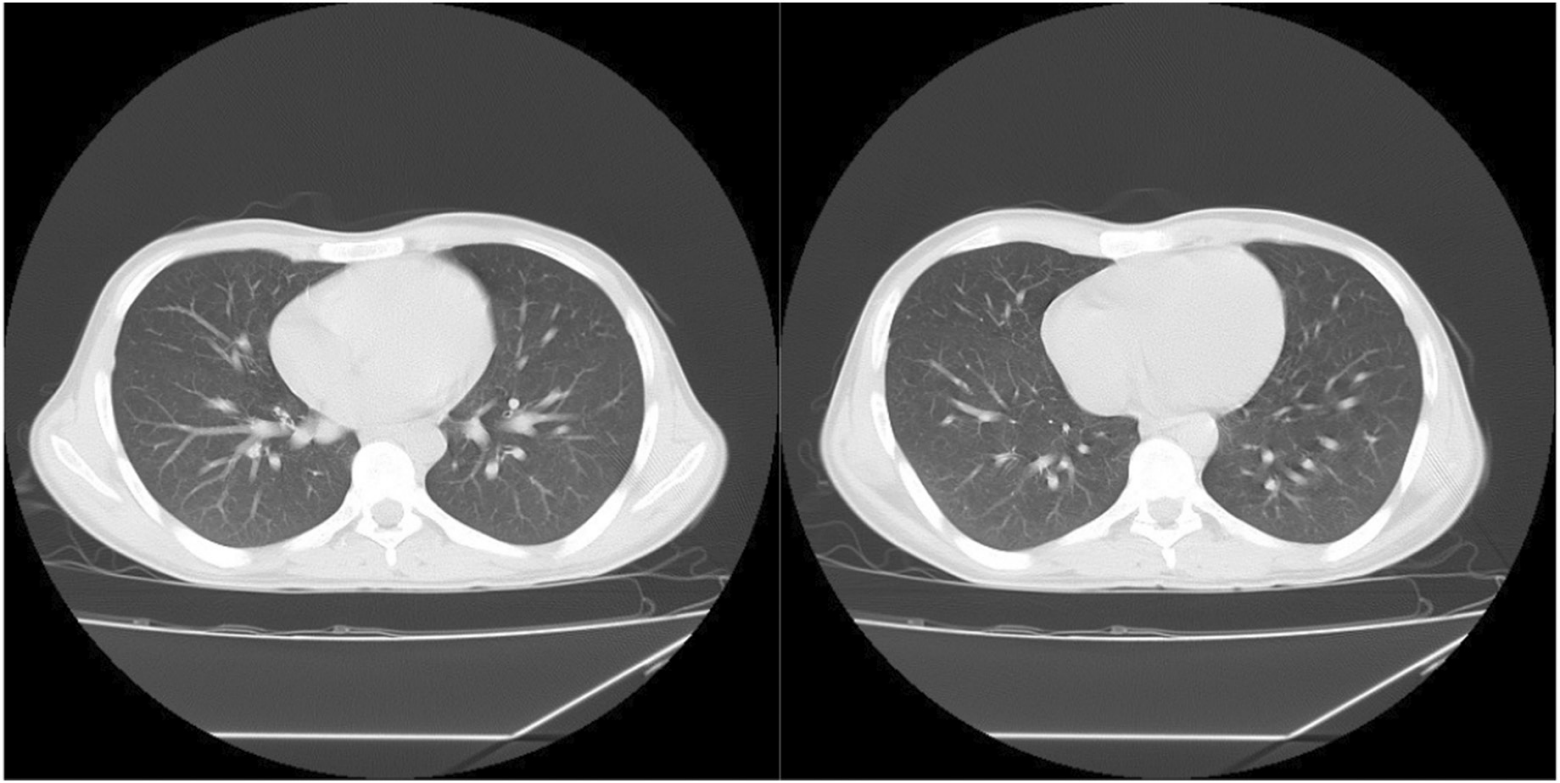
Chest CT scan. An 8‐mm subpleural nodule was observed in the lateral segment of the lower lobe of the right lung (RLL).
